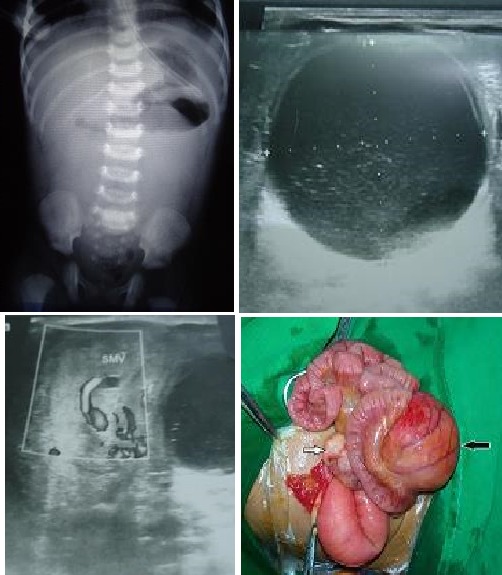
Figure 1: Showing plain x-ray, ultrasound abdomen, Doppler USG, and operative picture of jejunal duplication cyst (black arrow).The sub-hepatic mobile cecum and appendix suggested midgut malrotation (white arrow).
